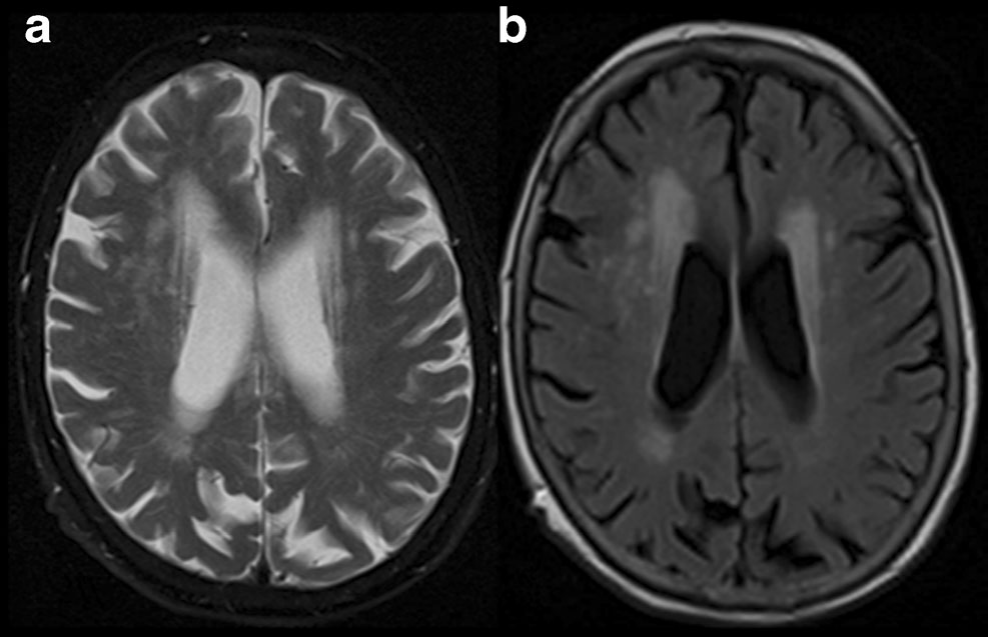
Sjogren’s syndrome. A 71-year-old female presented to hospital with unsteadiness, frequent falls and dementia with features of Sjogren’s syndrome. a & b axial T2W and FLAIR images of the brain show nonspecific patchy confluent hyperintensities in bilateral periventricular white matter
